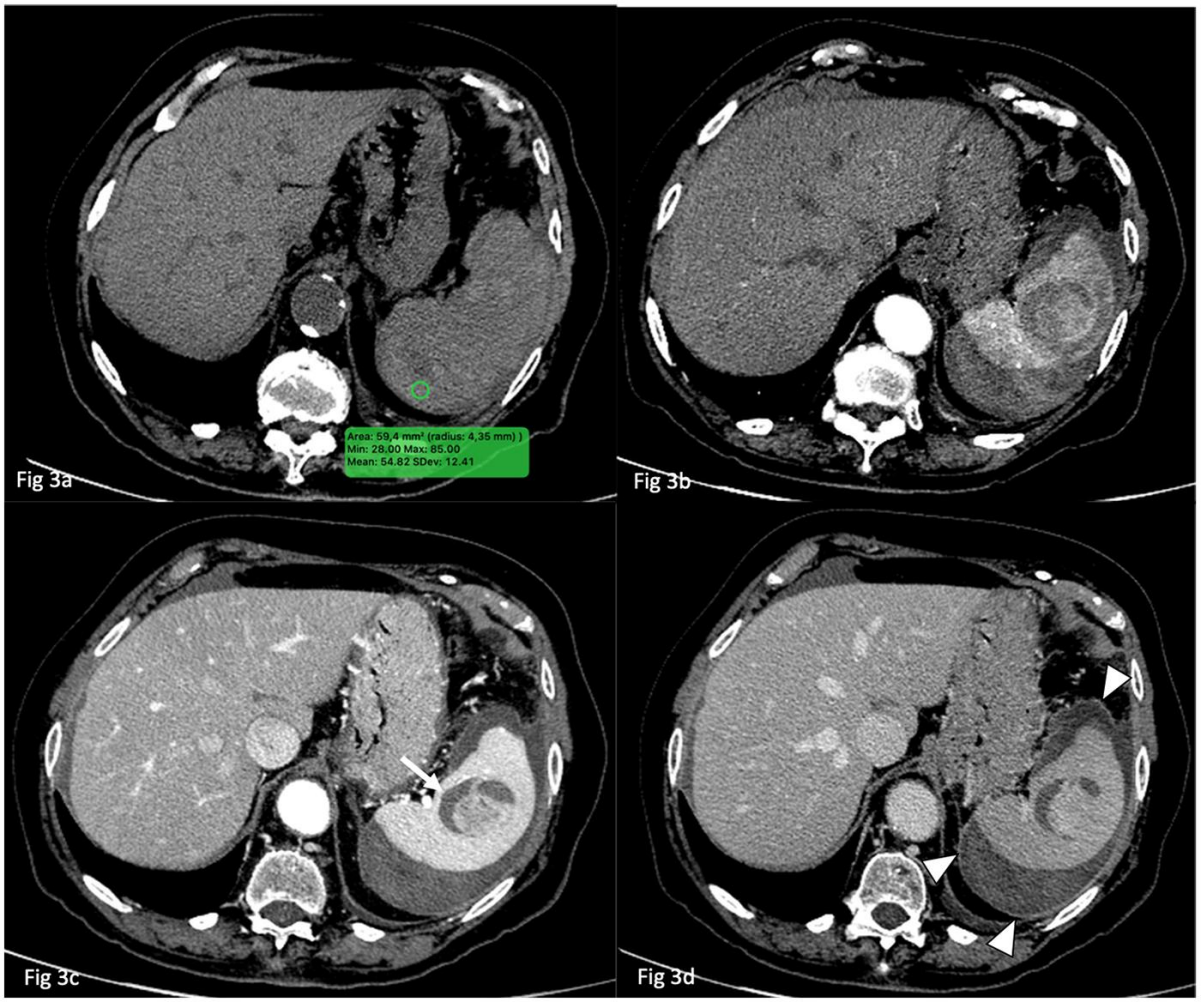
24 h follow-up CT scan. In (a) the unenhanced exam shows persistent perisplenic hyperdensity due to hemoperitoneum (*circle ROI*). In (b,c) the hemoperitoneum exhibits increased density heterogeneity due to blood degradation products in different phases of hemoglobin breakdown. The hypervascular central splenic lesion and peripheral hypodense halo (*arrow*) remain stable. There are still no signs of active bleeding but in in the late-phase CT axial scan (d), compartmentalization of hemoperitoneum is noted with the formation of a delayed contrast-enhancing wall (arrowheads).
